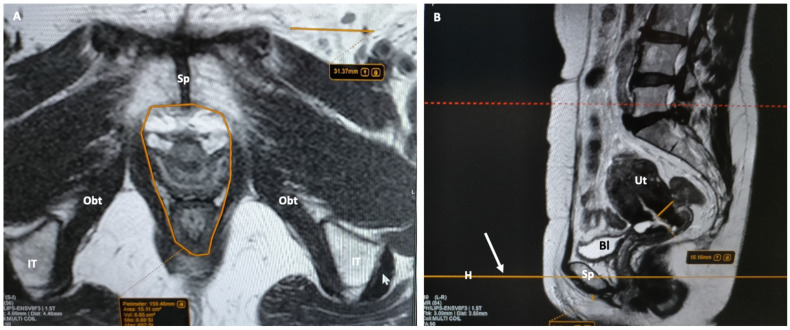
Method for free-hand tracing the area (orange contour) of levator ani hiatus on axial T2-weighted MR image (A) by selecting the scan plane on midsagittal image (B) and taking the middle of the long axis of pubic bone as reference (arrow). Sp: symphysis pubis; IT: ischial tuberosity; Obt: obturator muscle; Bl: bladder; Ut: uterus; H orange line: horizontal scan plane corresponding to (A).
